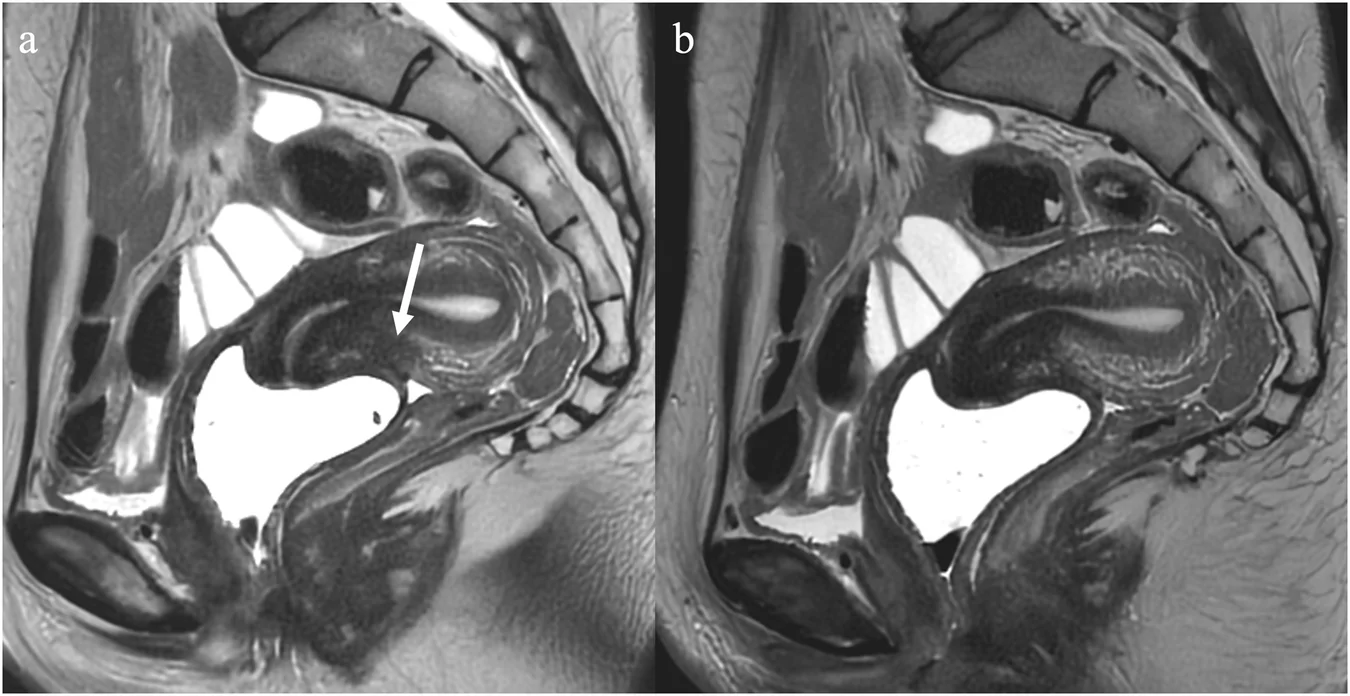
Uterine contraction in a 21-year-old woman with chronic catamenial pelvic pain. a, b Sagittal T2-weighted sequences at two different times. The initial MR images show a T2-hypointense focal thickening (arrow) of the torus uterinum. The second image shows the absence of abnormality of the torus corresponding to complete resolution of uterine contraction
